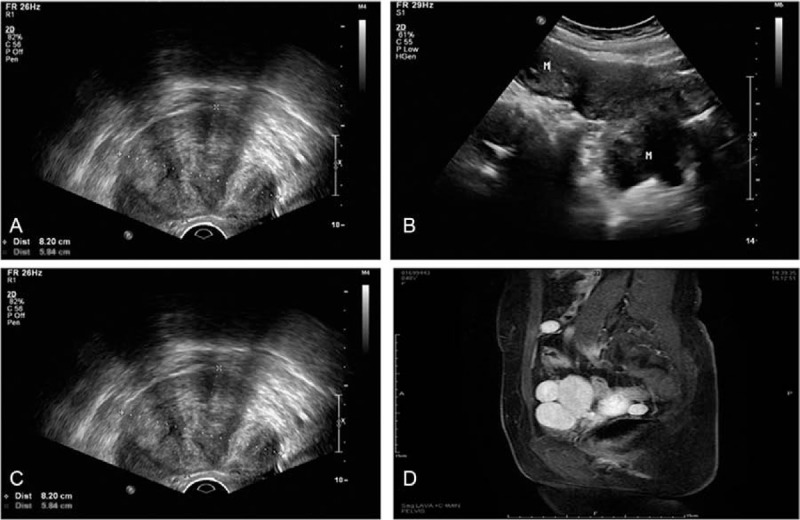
Ultrasound and MRI images of Case 3. A, B, Ultrasound showed multiple hypoechoic nodules in the uterus. C, Ultrasound showed a hypoechoic nodule in uterine rectal fossa, suggesting subserosal fibroids. D, Contrast-enhanced magnetic resonance imaging suggested multiple uterine fibroids and subserosal fibroids.
